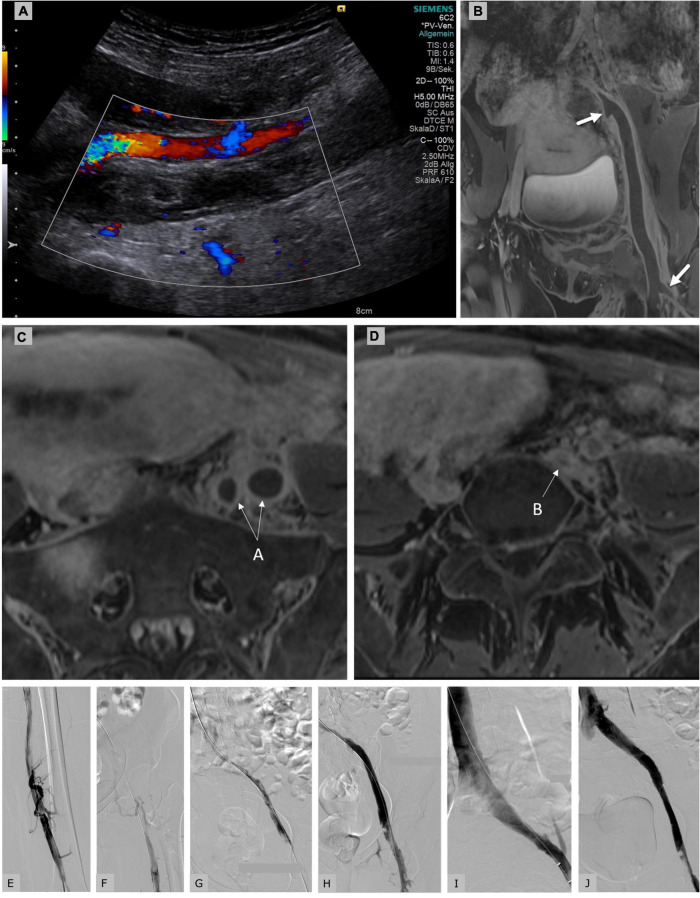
Duplex sonography (A) and MR-phlebography (B) of a 31-year-old female patient 10 days postpartum showing an iliofemoral thrombosis with involvement of the internal iliac vein (proximal arrow) and the deep femoral vein (distal arrow) down to the left popliteal vein. Clinically the left leg was tender and cool with slight sensible, but no motoric impairment; imminent phlegmasia was suspected. The patient was placed on a therapeutic dose of intravenously administered unfractionated heparin (Liquemin^®^, Drossapharm, Basel, Switzerland) which was adjusted according to repeated aPTT measurements. In the absence of clinical signs of bleeding and with a hemoglobin level within the normal range, catheter-directed thrombolysis (CDT) was performed via transcutaneous access of left occluded popliteal vein. After a bolus of 10 mg, a continuous infusion of alteplase (Actilyse^®^, Boehringer Ingelheim, Basel, Switzerland) was initiated at 2 mg/h for 5 h, and then reduced to 1 mg/h for 10 h. A total dose of 30 mg alteplase was applied. Insertion of an inferior vena cava filter was rejected after interdisciplinary discussion. Breast-feeding was paused for 24 h, but breast milk was collected before CDT to be fed to the infant later. aPTT levels were always documented to be within the therapeutic range (64–85 s) that is 1.5–2.5 times more than the baseline aPTT of 30 s. After discontinuation of thrombolysis the patient was placed on anticoagulation with subcutaneously administered enoxaparin 0.9 mg/kg bid (Clexane^®^, Sanofi, Vernier, Switzerland). (C) MR-phlebography of the same patient showing the transversal view of the enlarged thrombosed external and internal iliac veins (arrow A). (D) Proximal common iliac vein compression in the context of May-Thurner syndrome (arrow B). Initial phlebography of the partially occluded femoral veins (E) and of the occluded iliac veins (F) showing fresh thrombus. Control venography of our patient after catheter-directed thrombolysis (G), after Angiojet^®^ thrombectomy [Angiojet Zelante^®^ (8F), Boston Scientific, Larlborough, United States] (H), after balloon angioplasty (I), after stenting (Sinus obliquus 16/100, Optimed^®^, Ettlingen, Germany) of the left common iliac vein which was compressed in the context of underlying May-Thurner syndrome with residual thrombosis in the common femoral vein (J). The patient was discharged under anticoagulant treatment with enoxaparin 1.7 mg/kg qd for 3 months. No bleeding complications occurred neither during thrombolysis, pharmacomechanical thrombectomy nor during anticoagulation. No venous thrombembolic events occurred. Stent patency was confirmed sonographically after 3 months. After 4 months of treatment, the patient showed no signs or symptoms of PTS (Villalta-Score 0 point) and identical leg circumferences.
